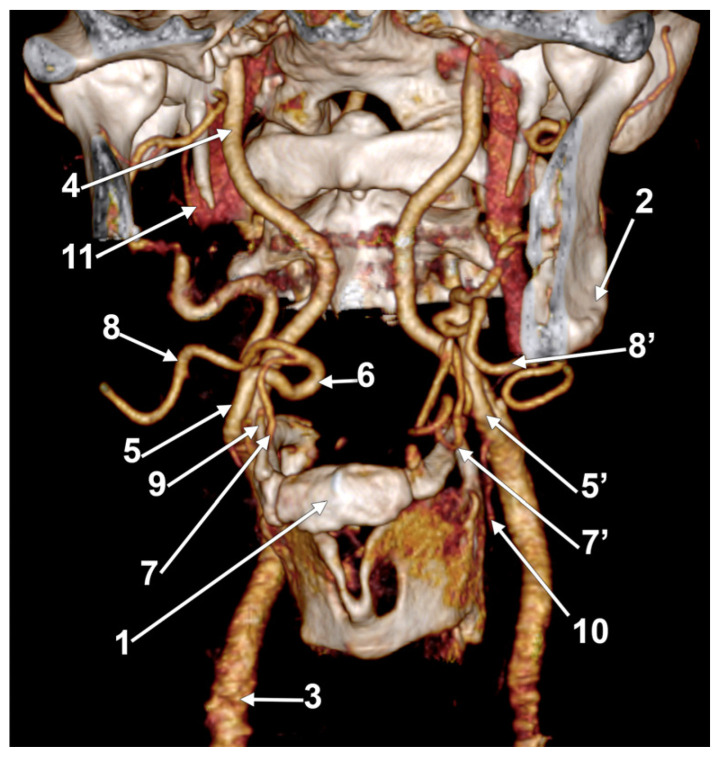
Right linguofacial trunk. Computed tomography angiogram. Three-dimensional volume rendering. Antero-inferior view. 1. hyoid body; 2. gonial angle; 3. right common carotid artery; 4. right internal carotid artery; 5, 5’. external carotid arteries; 6. linguofacial trunk; 7, 7’. lingual arteries; 8, 8’. facial arteries; 9. ossified ceratohyal; 10. superior thyroid arteryș and 11. internal jugular vein.
